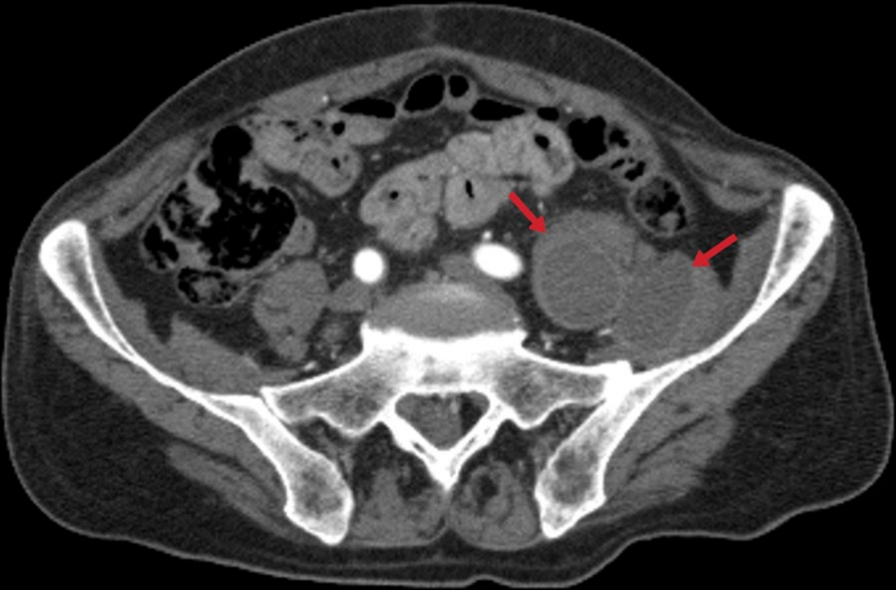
A contrast-enhanced CT image of a 69-year-old male demonstrates the left iliopsoas abscess (arrows)
